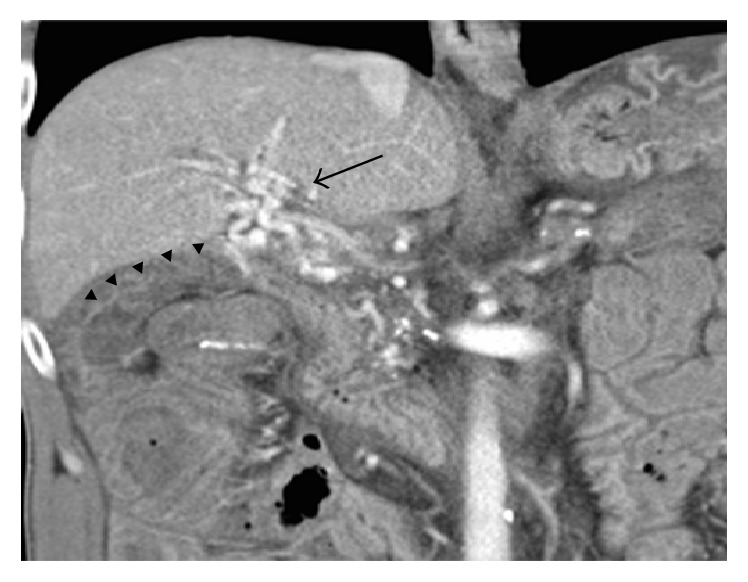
Coronal reformat of the portal venous phase of a multiphase CT liver examination shows an occluded main portal vein with cavernous transformation (arrow). The efferent limb of the hepaticojejunostomy (arrowheads) can be seen extending away from the lateral aspect of the venous collaterals.
